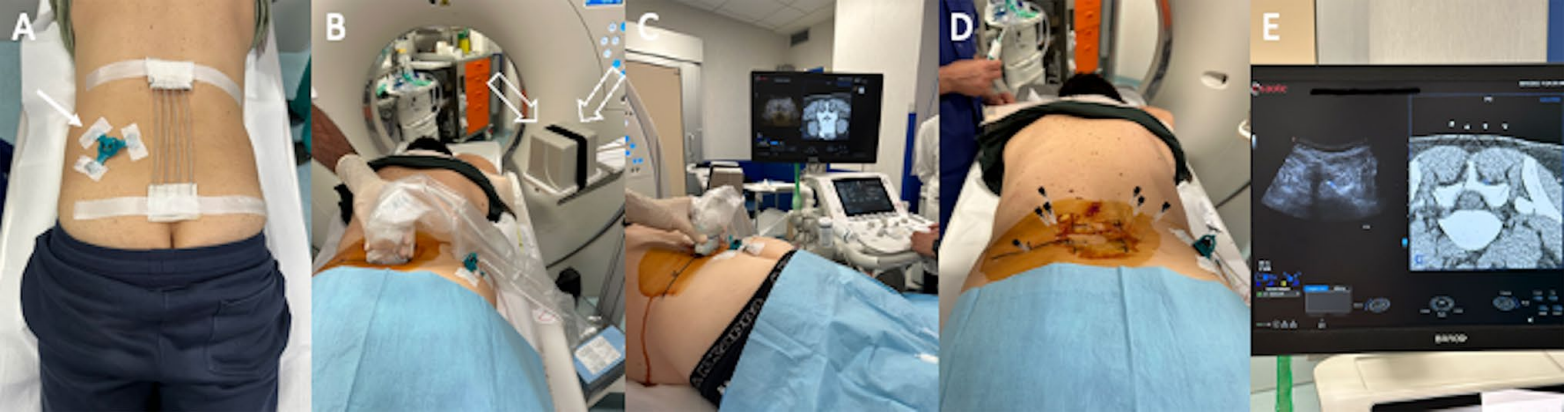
US/CT fusion imaging guided lumbar intradiscal O2-O3 injection. An external fiducial marker was positioned close to the relevant area (arrow, A). After disinfection, US probe was placed on the skin to merge images with CT scan (B, C) that has been already loaded onto the US machine equipped with a GPS-sensor (void arrows, B). Six needles were introduced into each facet joint at L3-L4, L4-L5, and L5-S1 levels, and one needle was introduced through a paravertebral oblique postero-lateral in-plane approach to reach the center of the herniated disc (D). The navigation system allowed real-time fusion of CT and US image with perfect superimposition of the posterior vertebral arch (E)
